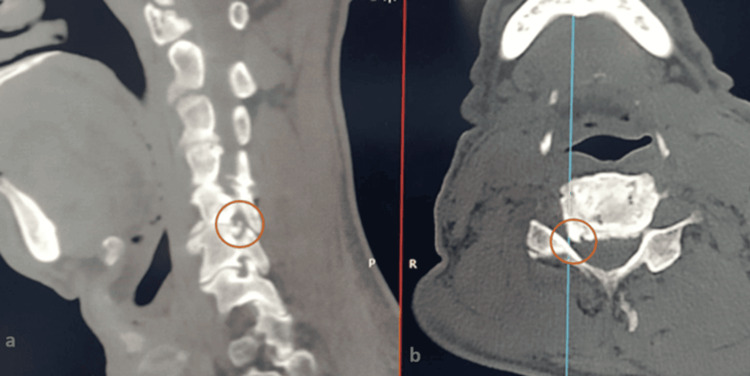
Osteophyte formation is visible in a CT scan of C5/C6 cervical disc degeneration (a) Sagittal and (b) axial slices (marked by the orange circle).
